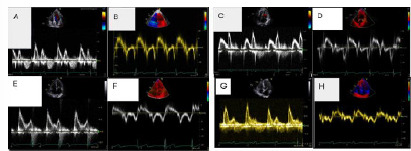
Demonstration of the mitral valve inflow velocities (MVI) and tissue Doppler indices (TDI) for respective grades of diastolic dysfunction. Image A and B are the MVI and TDI for a patient with grade 0 (normal diastolic function), image C and D for grade I, Image E and F for grade II, and image G and H for grade III.
